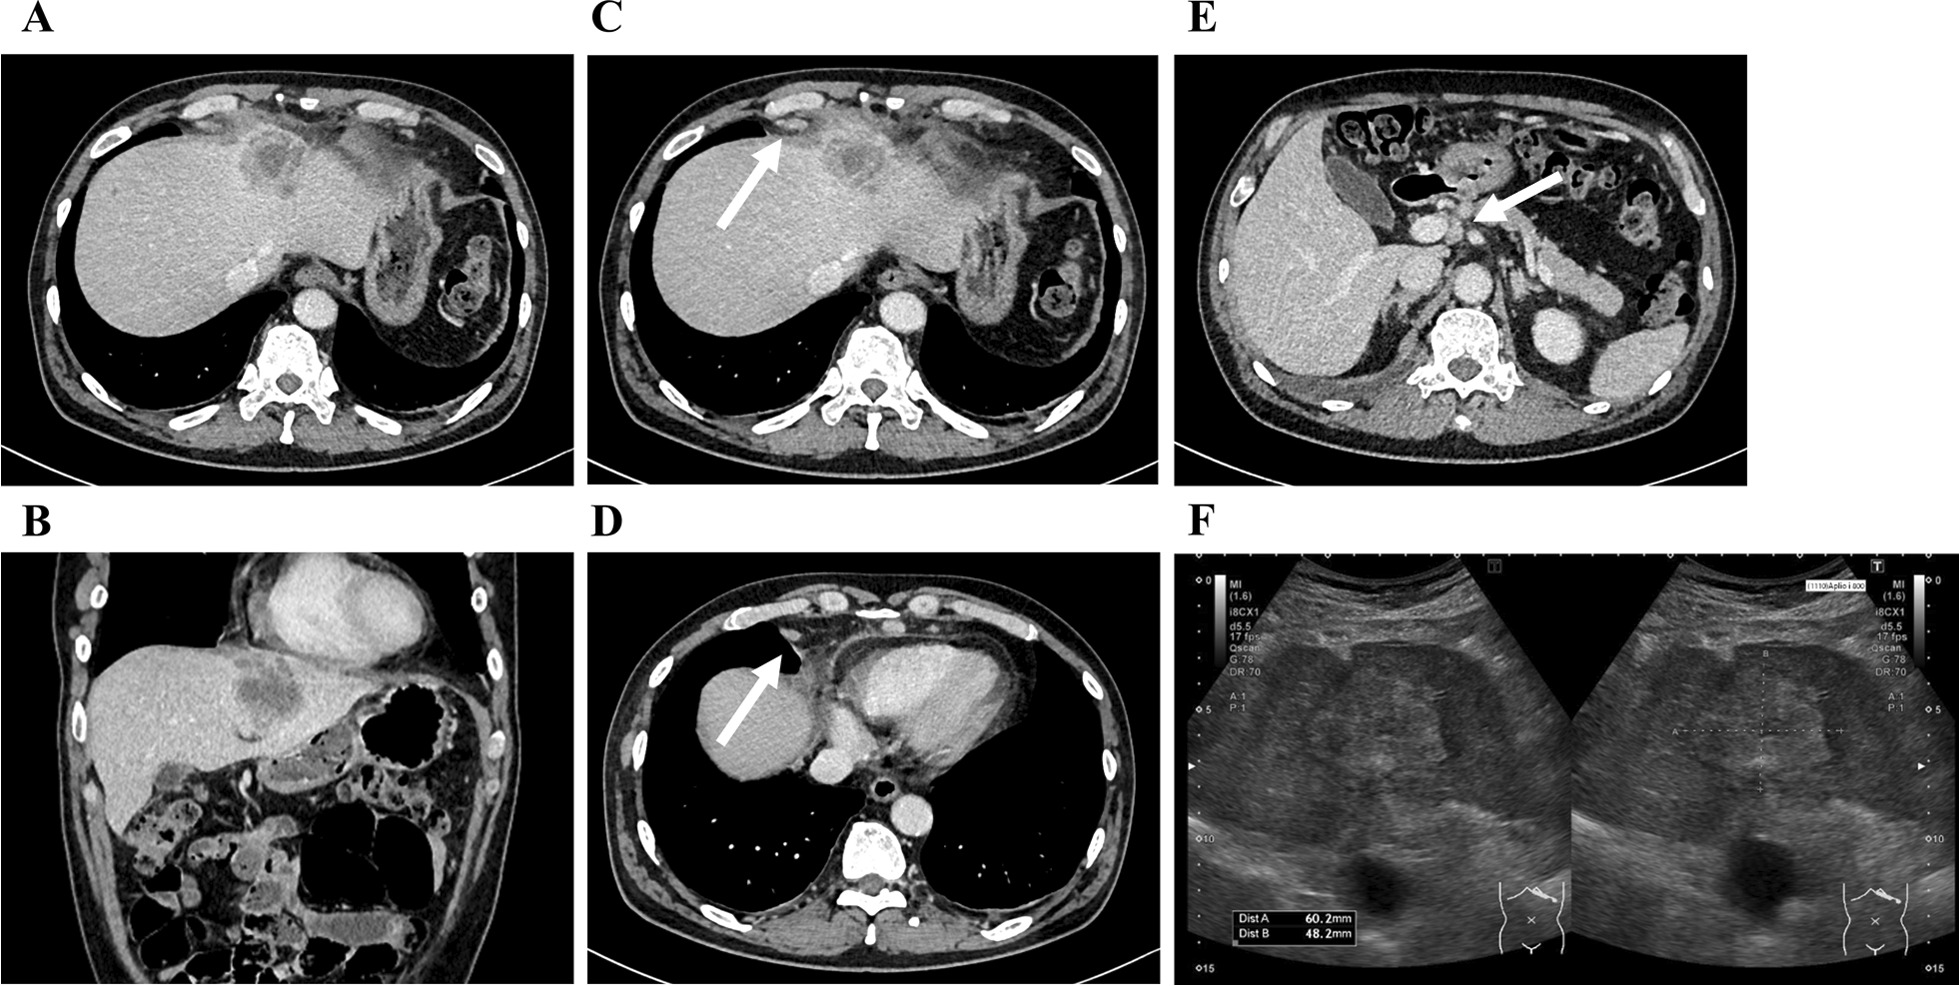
Abdominal contrast-enhanced computed tomography and ultrasonography on admission. A, B Abdominal contrast-enhanced computed tomography shows a tumor with a low-density area in the left lobe of the liver (A axial image, B sagittal image). C–E Axial images of the abdominal contrast-enhanced computed tomography shows swelling in multiple lymph nodes (white arrows). F Abdominal ultrasonography shows a hyperechoic solid tumor with 60.2 × 48.2 mm in diameter in the left lobe of the liver
